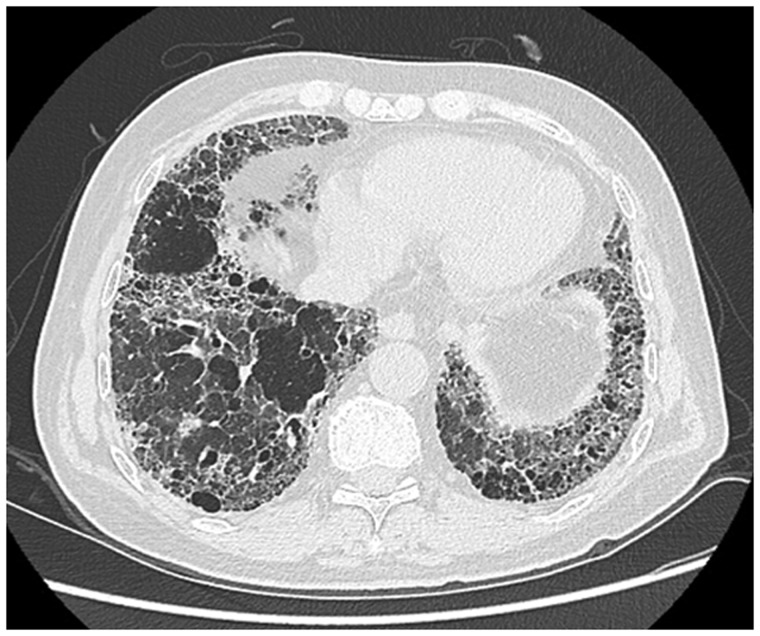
Idiopathic pulmonary fibrosis. High-resolution CT scan showing diffuse areas of interstitial thickening in both lungs with honey-combing and fibrosis in a case of biliary cirrhosis.
